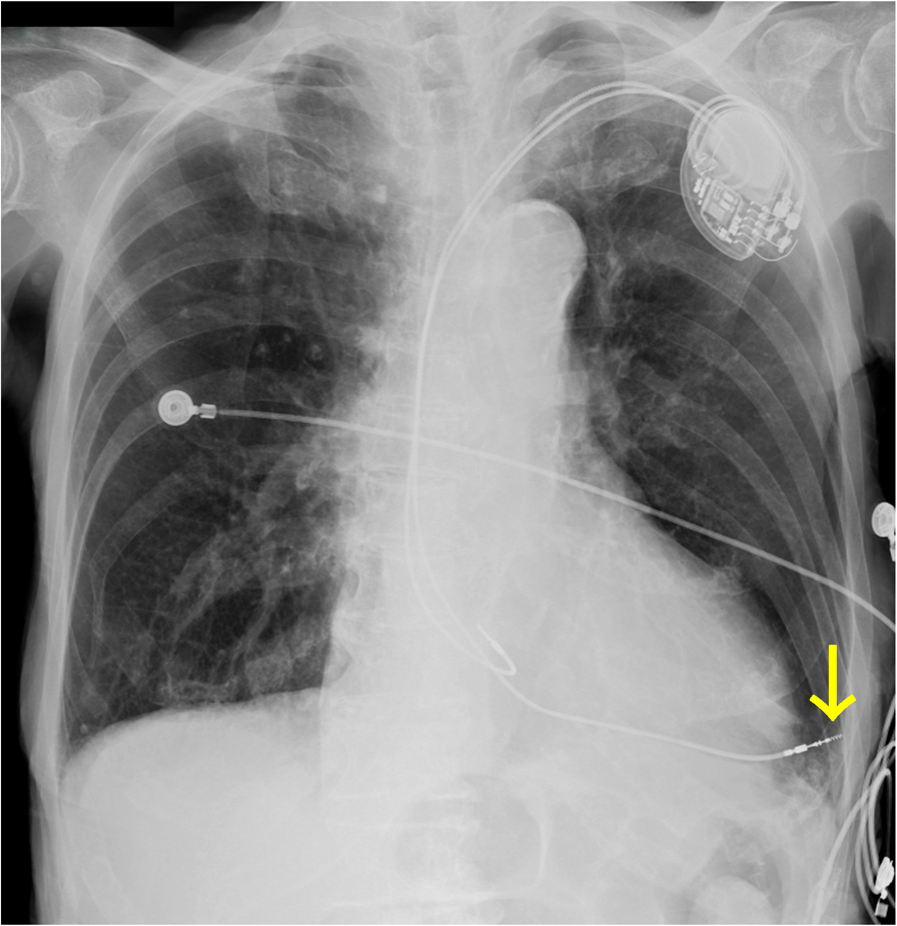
A preoperative chest radiograph. Chest radiograph showing the pacemaker lead perforating the right ventricle (yellow arrow)
